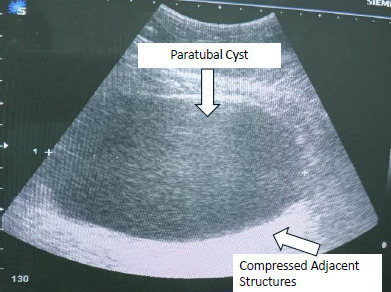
Abdominal ultrasound demonstrating a large right-sided unilocular adnexal mass, later confirmed to be a paratubal cyst, measuring 18 cm in its largest diameter.
